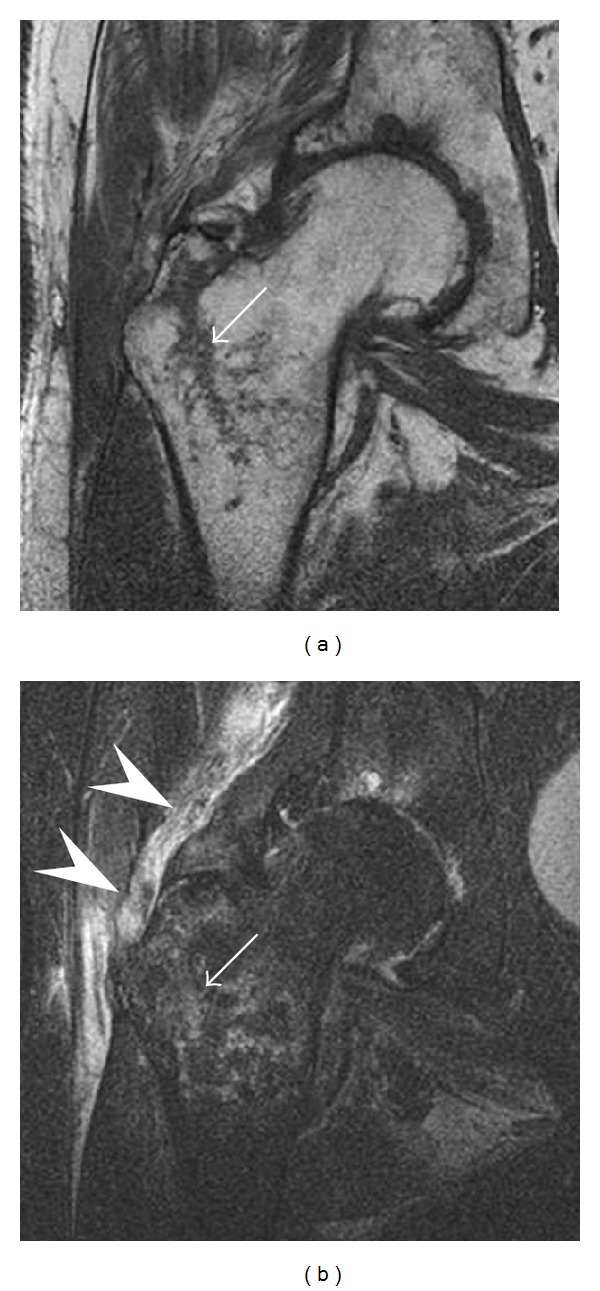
Partial osseous avulsion of the gluteal muscles at the greater trochanter in a 59-year-old man who presented with the right hip pain without a history of trauma. Lauenstein view and anteroposterior and radiographs (not shown) did not show an obvious fracture line or disruption of bony contours in the acetabulum or the right femoral neck. (a) Coronal T1-weighted MRI displays an incomplete fracture line extending partially from the greater trochanter (arrow). (b) Coronal short tau inversion recovery MRI shows heterogeneous hyperintensity in the same region (arrow) as well as hyperintensity within the gluteus medius and minimus muscles (arrowheads) consistent with tissue edema and hematoma.
